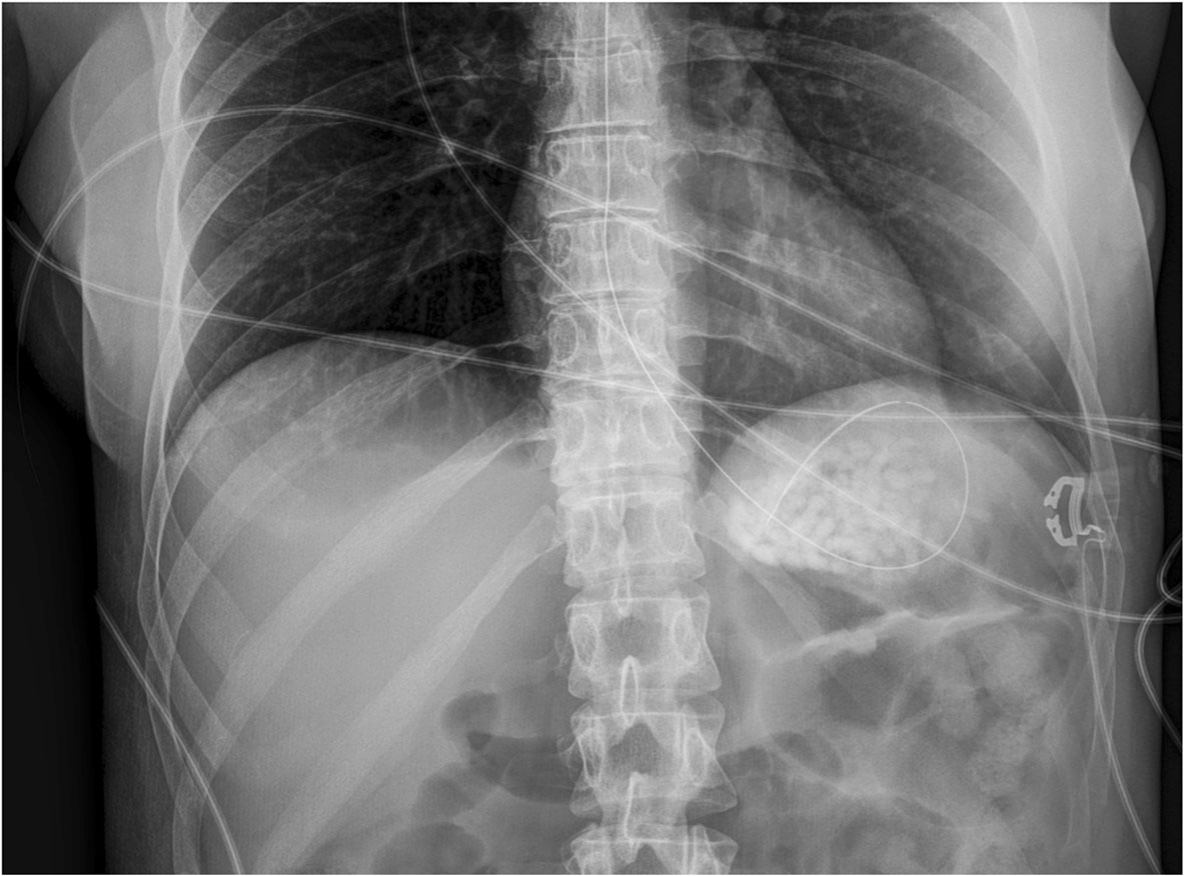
Abdominal x-ray confirming presence of gastric pharmacobezoar
